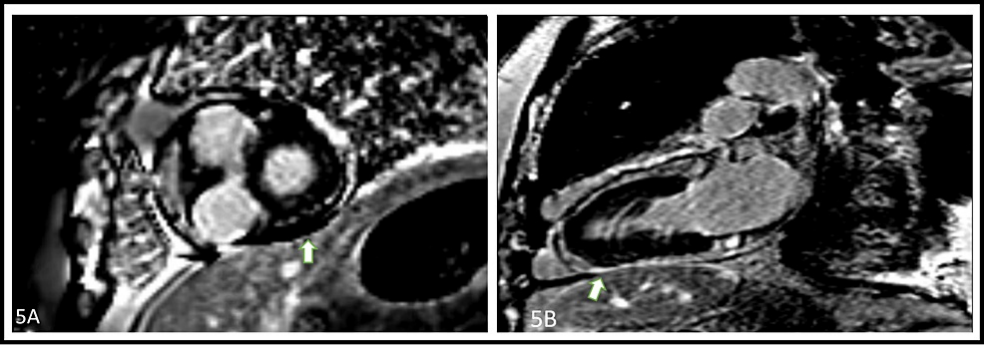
Repeat cardiovascular magnetic resonance imaging mono-images demonstrating limited to absent late gadolinium pericardial enhancement suggestive of limited pericardial inflammation
